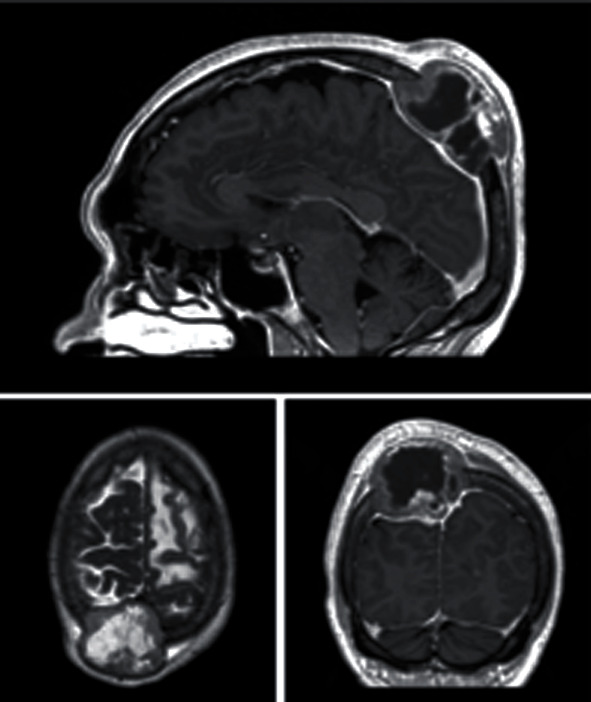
Images from brain MRI study at diagnosis.
